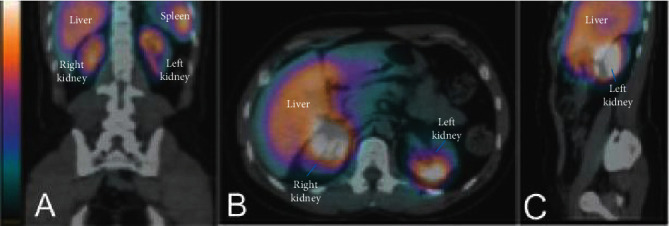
Representative images of SPECT/CT fusion in patient 2 at 2 hr after the infusion of ^99m^Tc-labeled BMDMCs showing uptake in the renal topography through different views: (A) coronal, (B) axial, and (C) sagittal. Increasing uptake is indicated by a color shift from blue to red to yellow to white.
